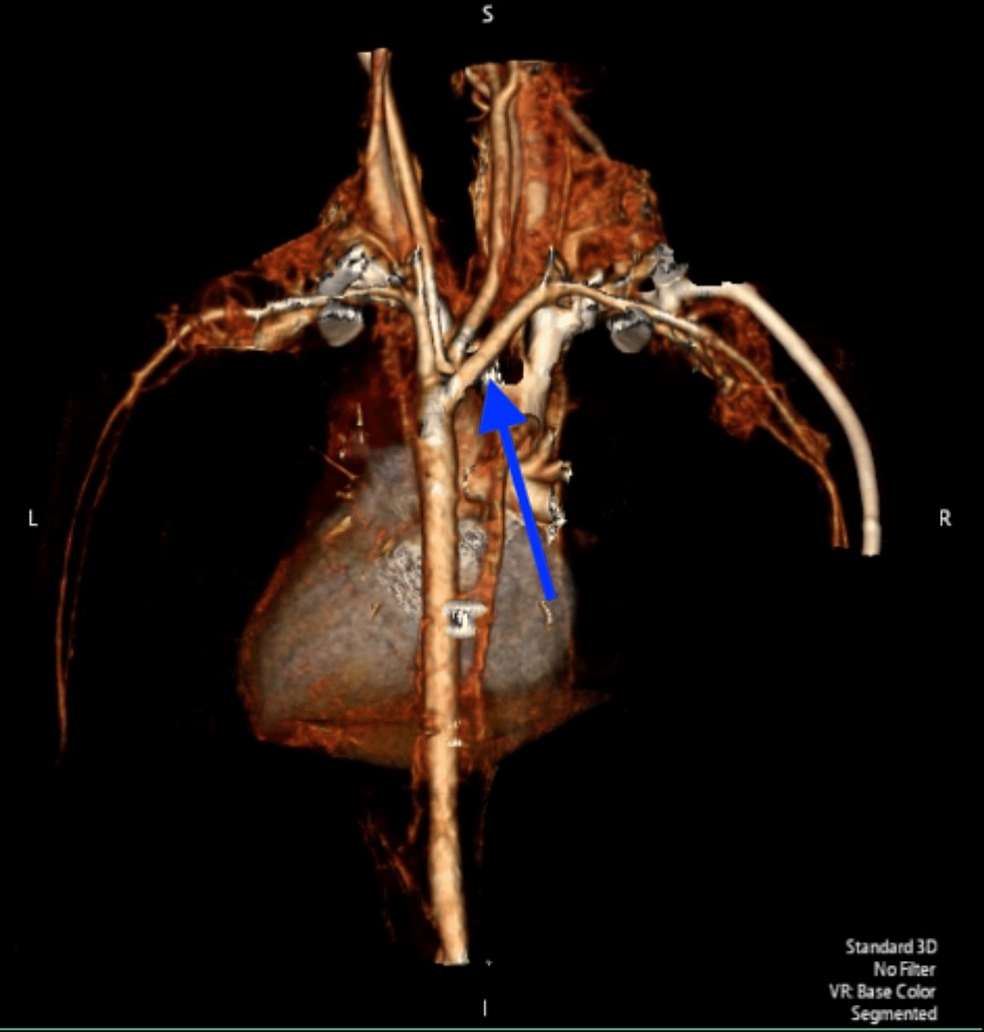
The 3D CTA shows a posterior view of the left-sided aortic arch with a common origin for right and left common carotid arteries as well as an ARSA arising directly from the aortic arch. 3D: Three dimensional, CTA: Computed tomography angiography, ARSA: Aberrant right subclavian artery
